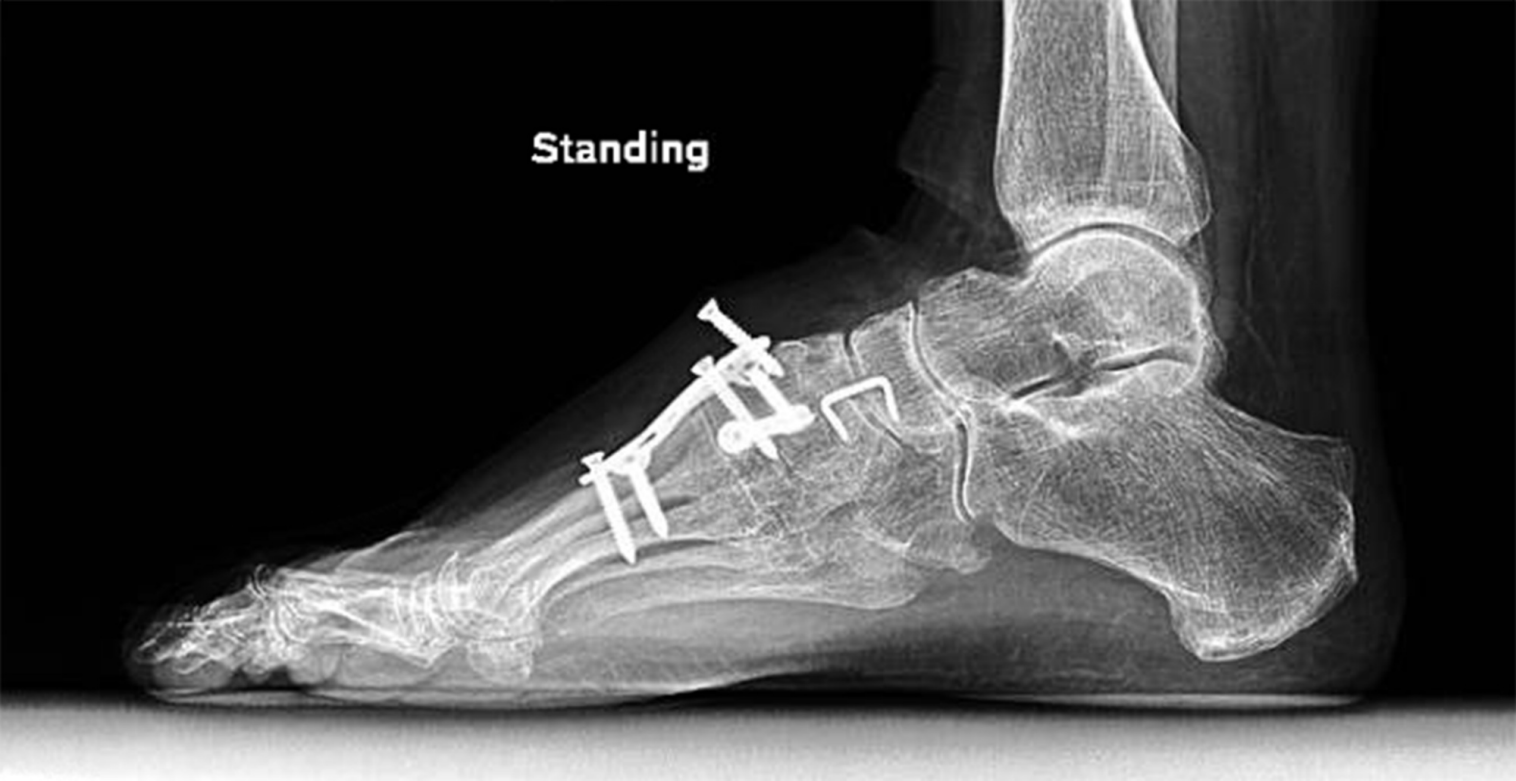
Eight-month follow-up radiograph shows the posterior-most fixation screw backed out of the dorsal metatarsophalangeal plate approximately 9 mm
